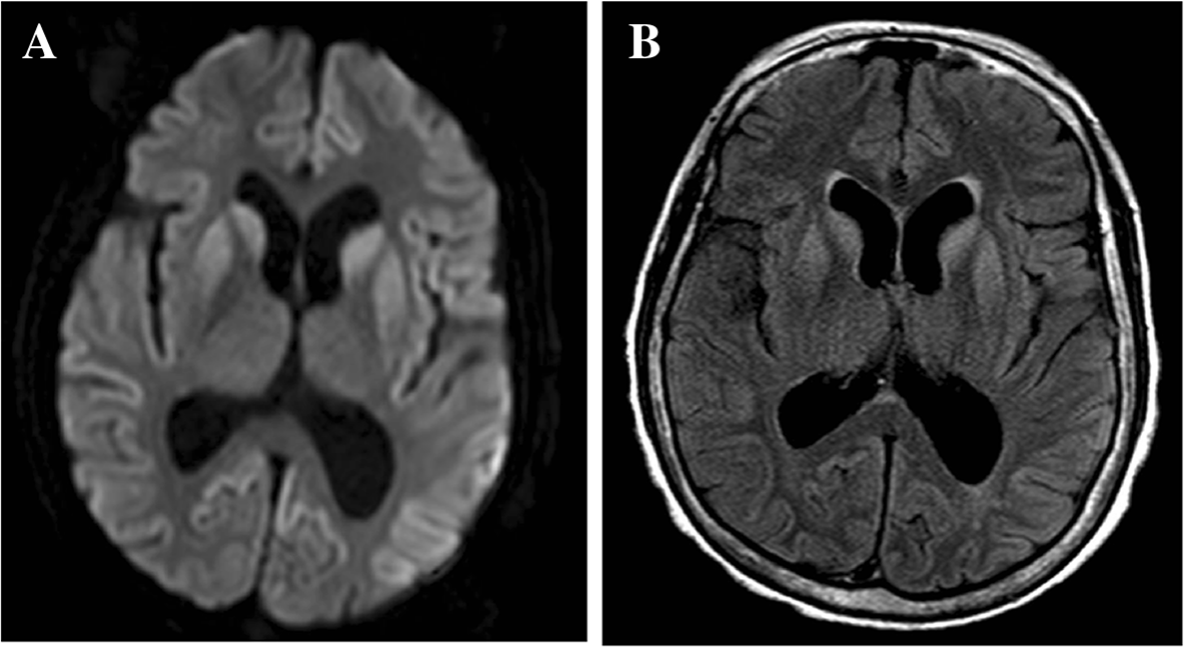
Patient MRI scans. Bilateral high signal intensities in the cortex, caudate nucleus, and putamen on DWI (A) and FLAIR (B).
